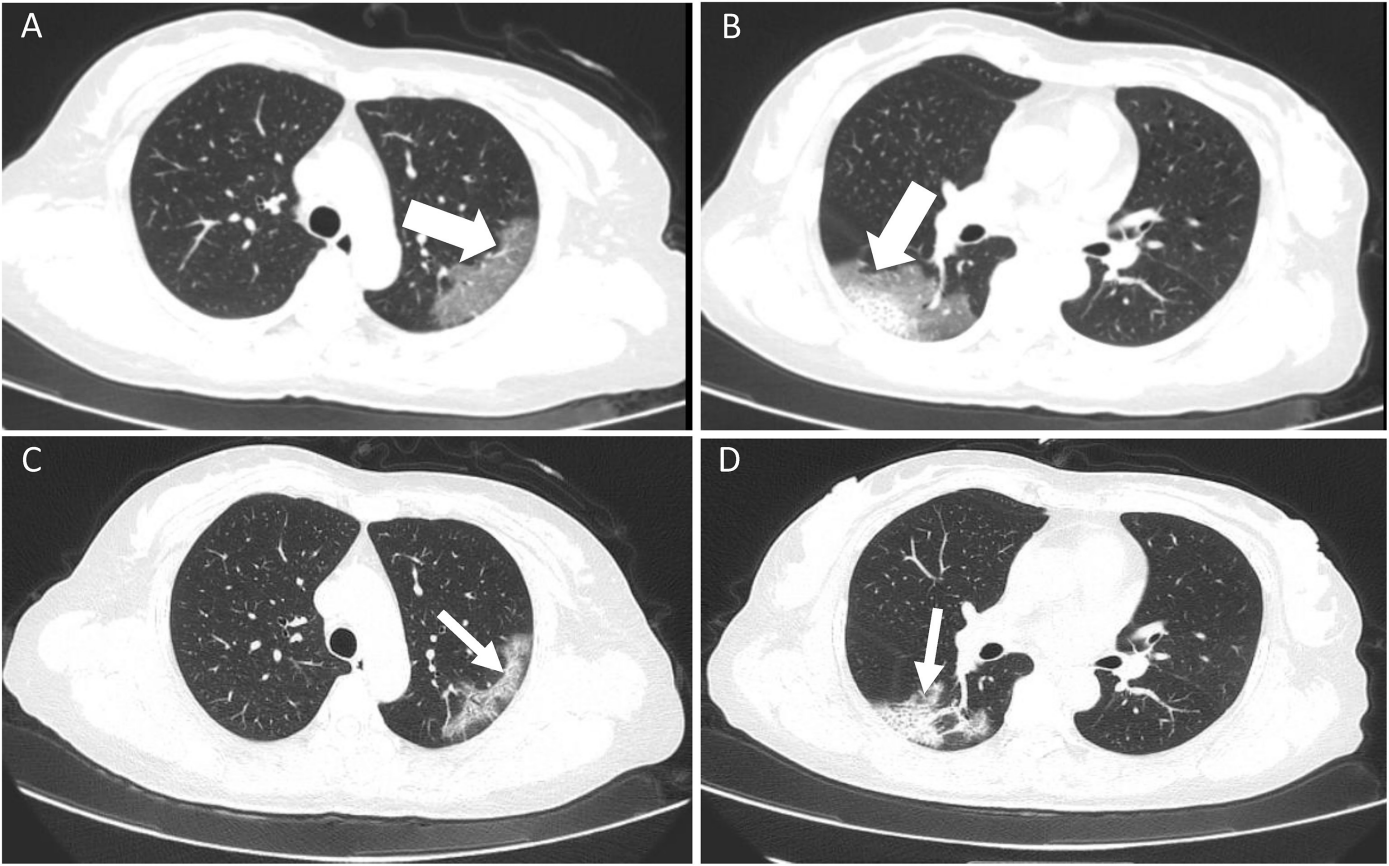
A 52-year old female with confirmed COVID-19 infection. Patient had close contact with a confirmed case and the onset symptom of fever. (A,B) Initial CT scan (performed on February 5 2020) showed bilateral GGO and mixed GGO and consolidation (white thick arrow) with an easily differentiated margin. The viral load (*Ct* value) was 38.65. (C,D) The follow-up CT scan (performed on February 9 2020) showed an improvement change. All the lesions had been absorbed (white fine arrow).
